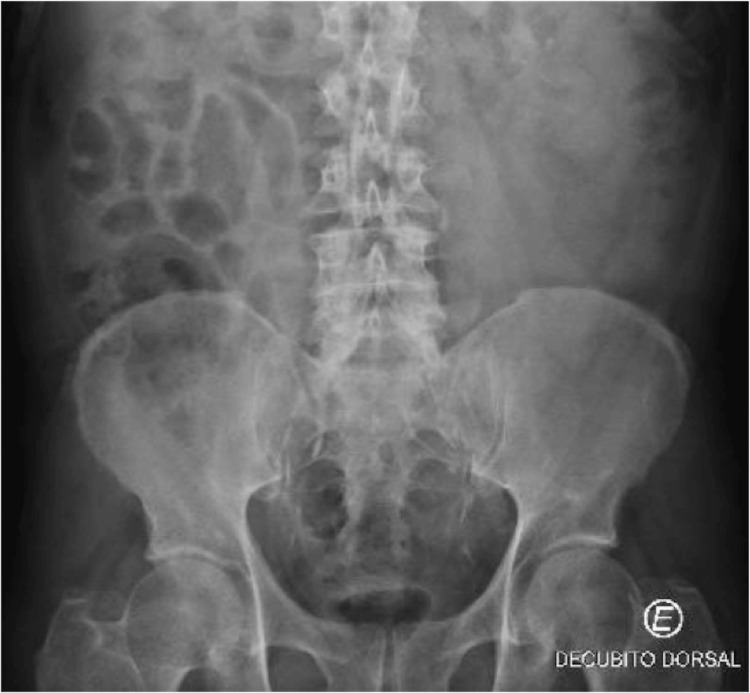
Abdominal x-ray with aerocolia
